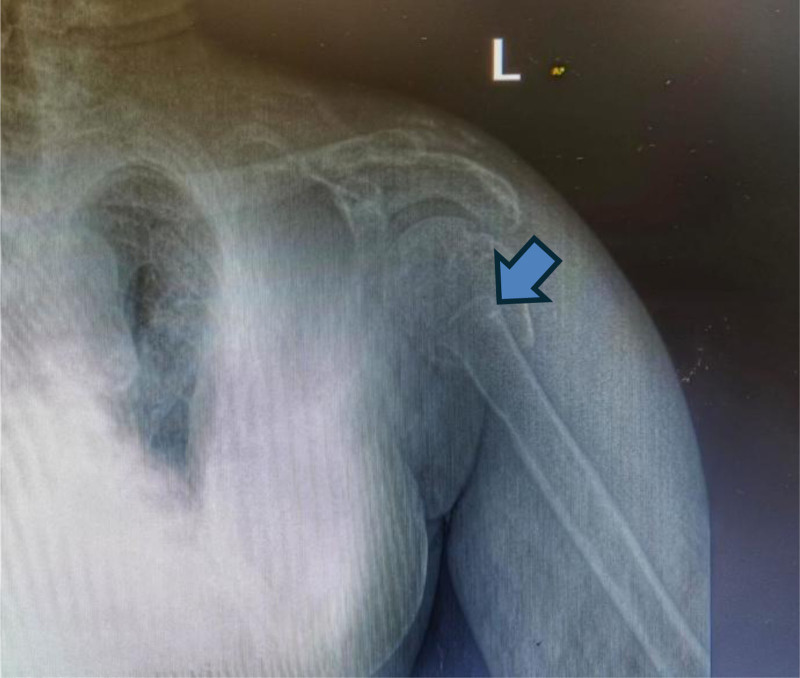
X-ray left shoulder joint showing left humerus fracture pointed by blue arrow.
